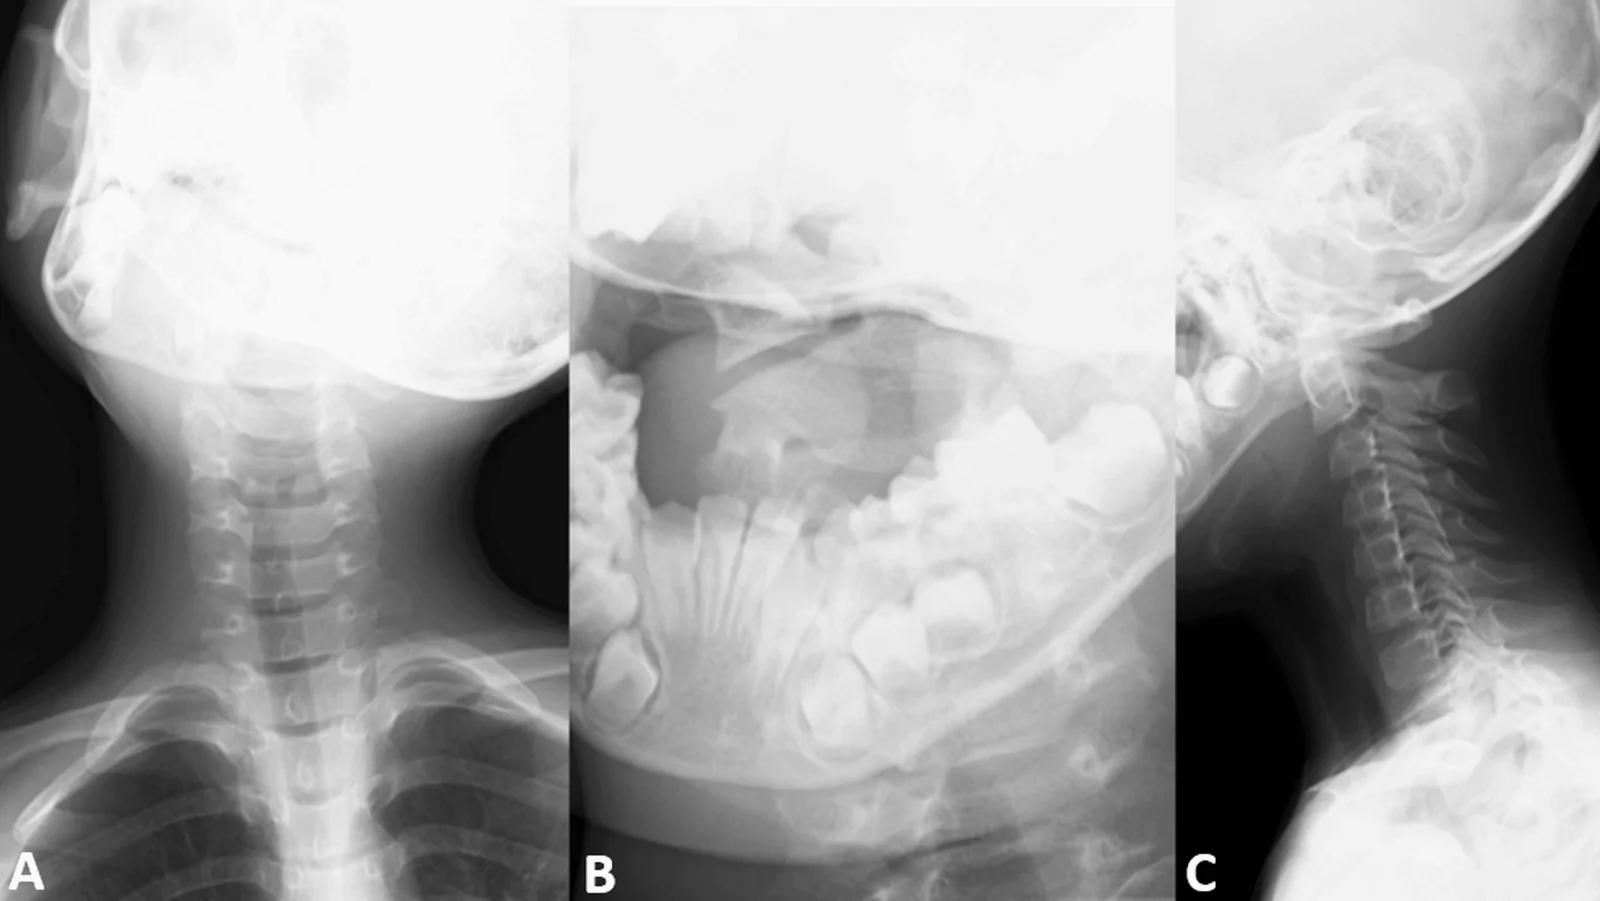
Initial plain radiographs. (A) Anteroposterior view. (B) Open-mouth odontoid view. (C) Lateral view.
